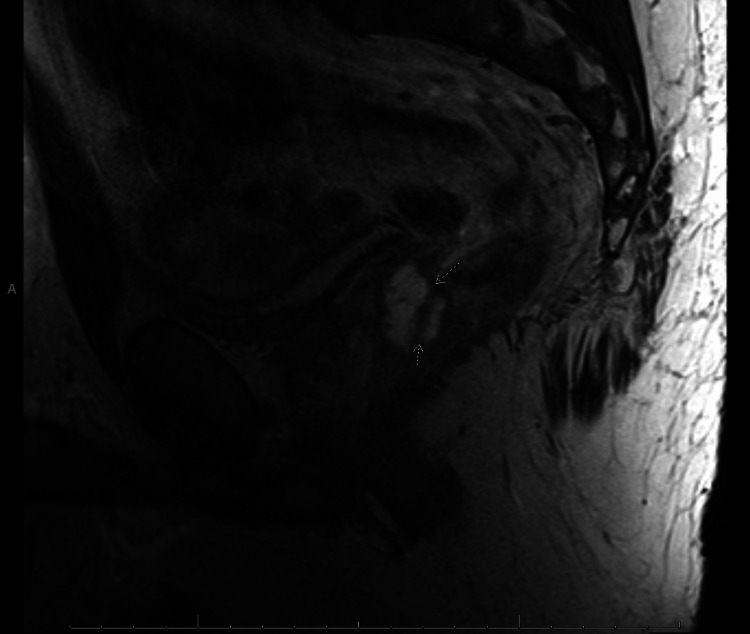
T2-weighted sagittal MRI (image taken laterally to Figure 1) with arrows indicating spaceOAR (hypointense) dissection of the rectal wall (hyperintense line)
